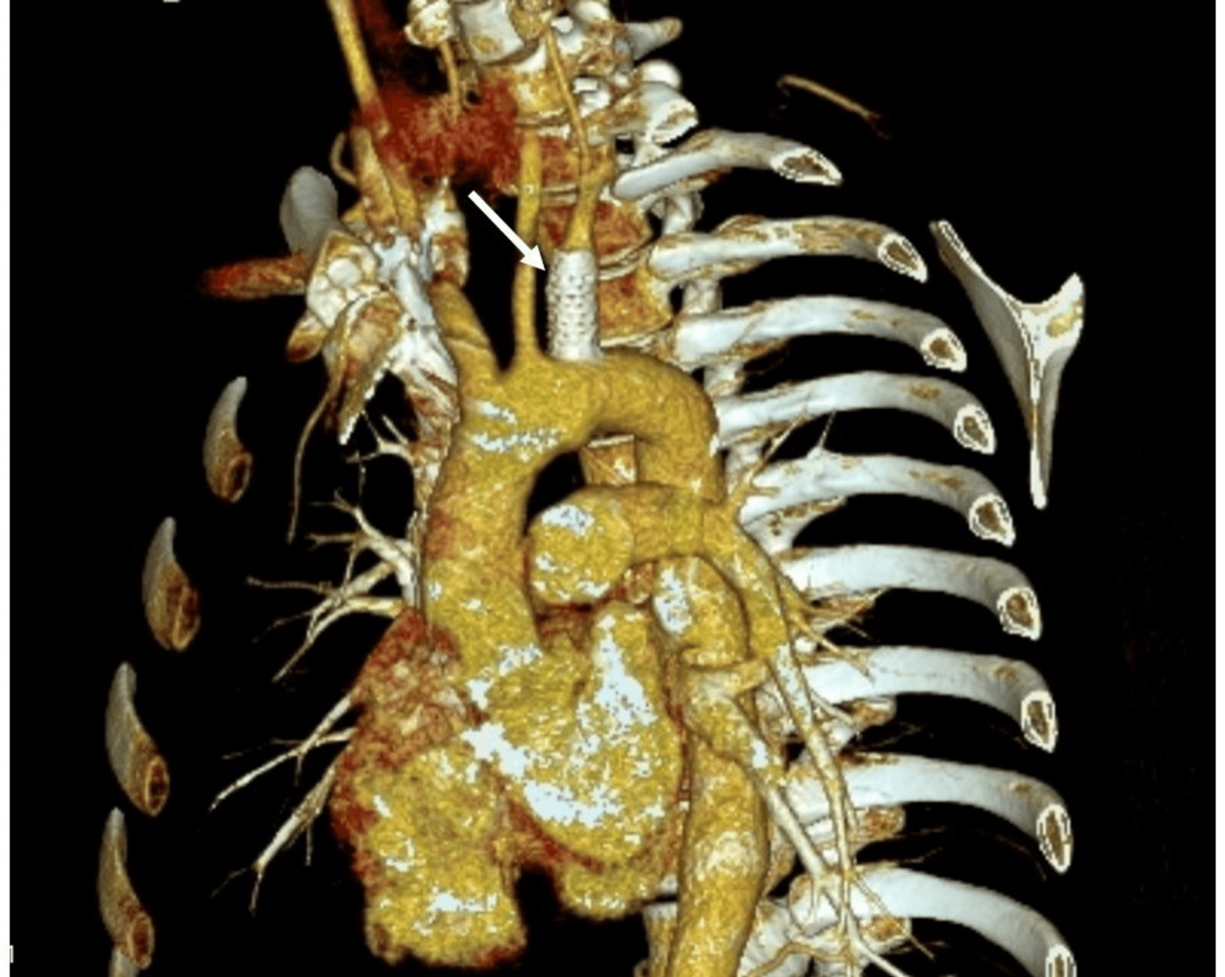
CT angiography depicting patent stent-graft covering subclavian pseudoaneurysm (white arrow) Three-dimensional volume-rendering reconstruction
